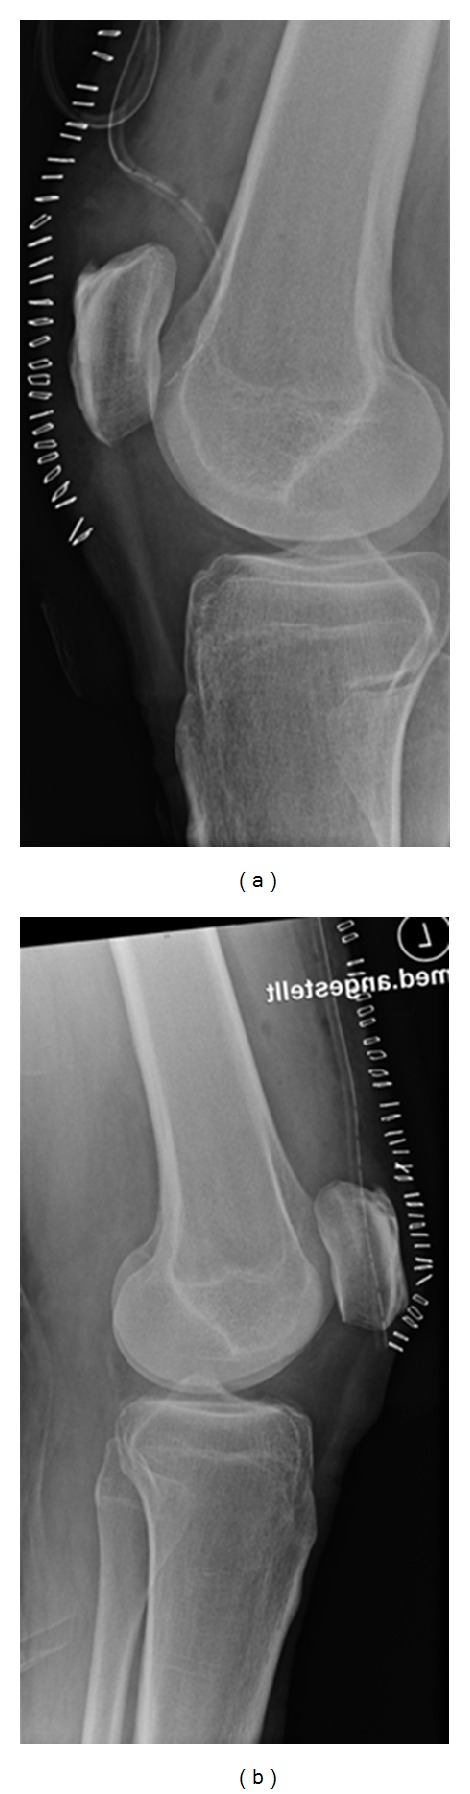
Postoperative radiographies demonstrate regular positioning of the patellae.
